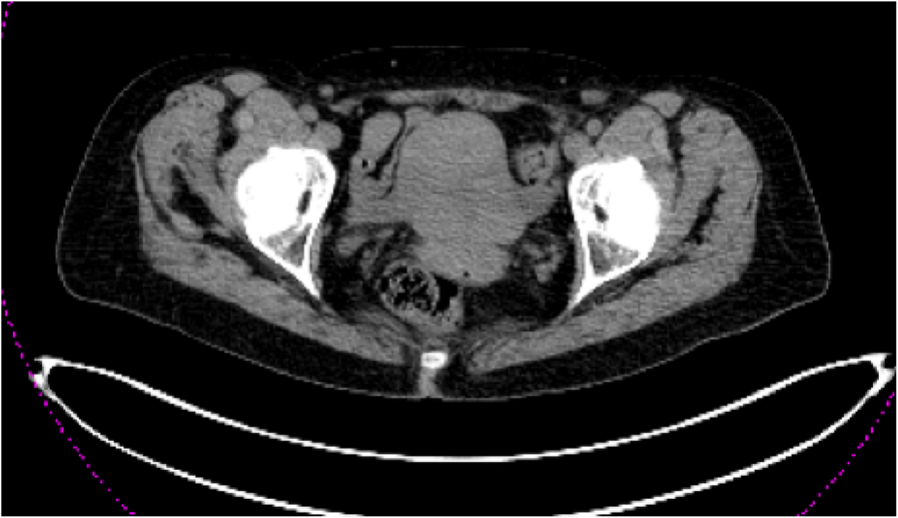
Computed tomographic scan
